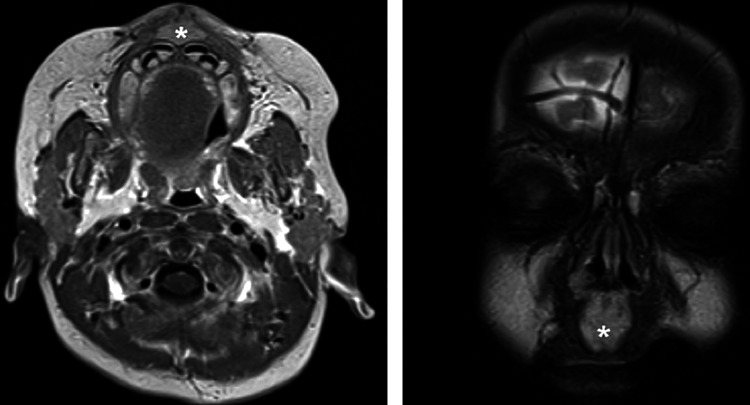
MRI of the head (left) with and (right) without contrast. Findings are consistent with proliferative hemangioma of the premaxilla (*), at the level of the nasal labial folds without the involvement of the oral cavity.
